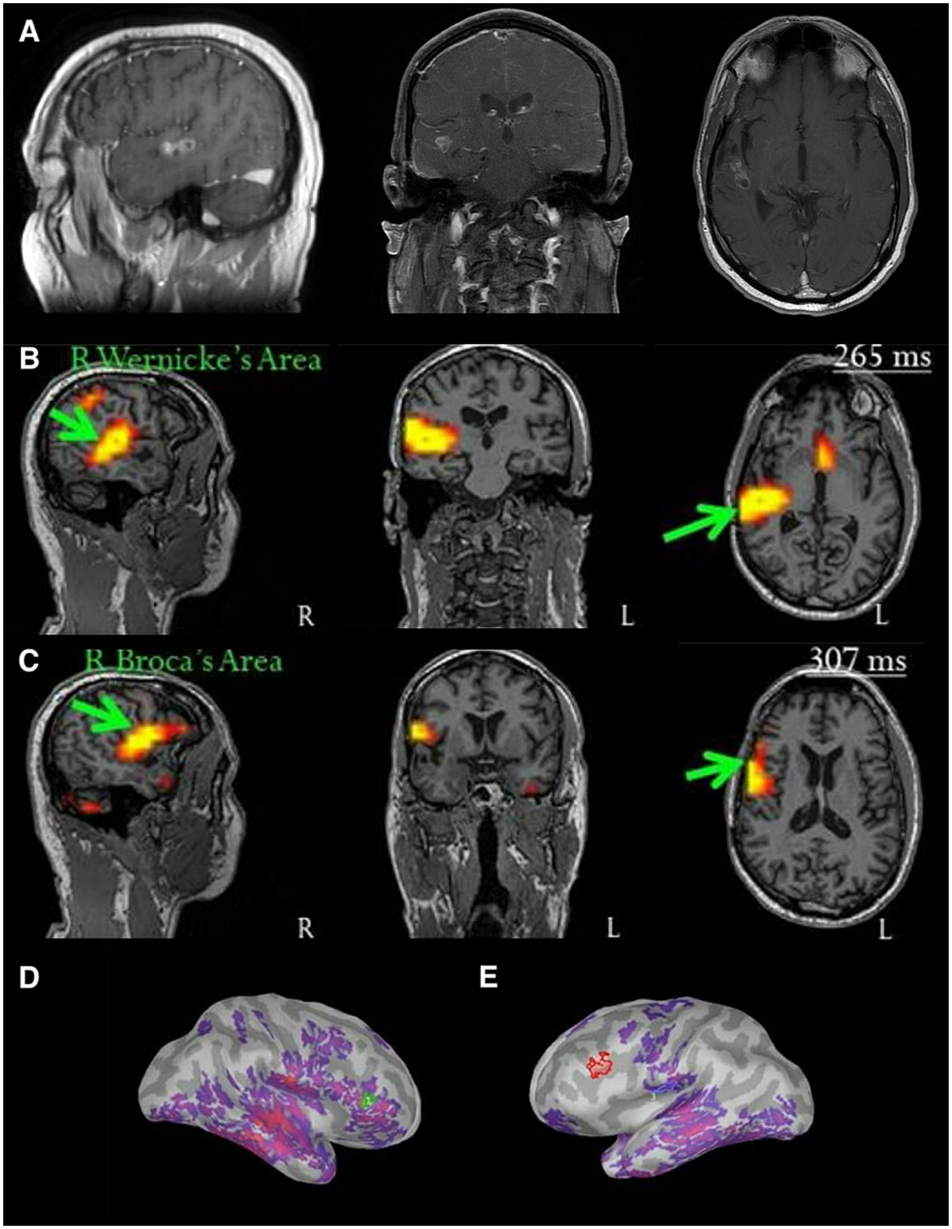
Multimodal preoperative workup for a right temporal glioblastoma. All scans are displayed according to radiologic convention. (A) Structural MRI demonstrating nodular enhancement near the prior resection cavity at the superior aspect of the right temporal lobe, (B) magnetoencephalography (MEG) demonstrating activation of the right posterior temporal lobe, consistent with Wernicke’s area, using a word comprehension paradigm, (C) MEG demonstrating activation of the right frontal lobe, consistent with Broca’s area, using a naming task, and (D,E) show images rendered from language activation patterns on MEG in the right and left hemispheres, respectively, superimposed on the patient’s structural MRI.
